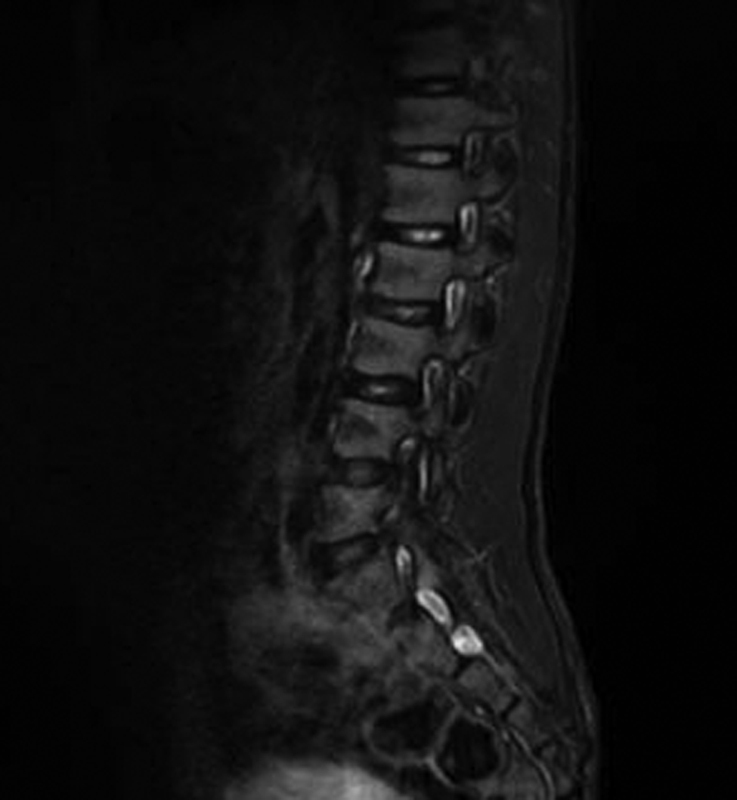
Spine magnetic resonance imaging showing S2 periapical cyst.
